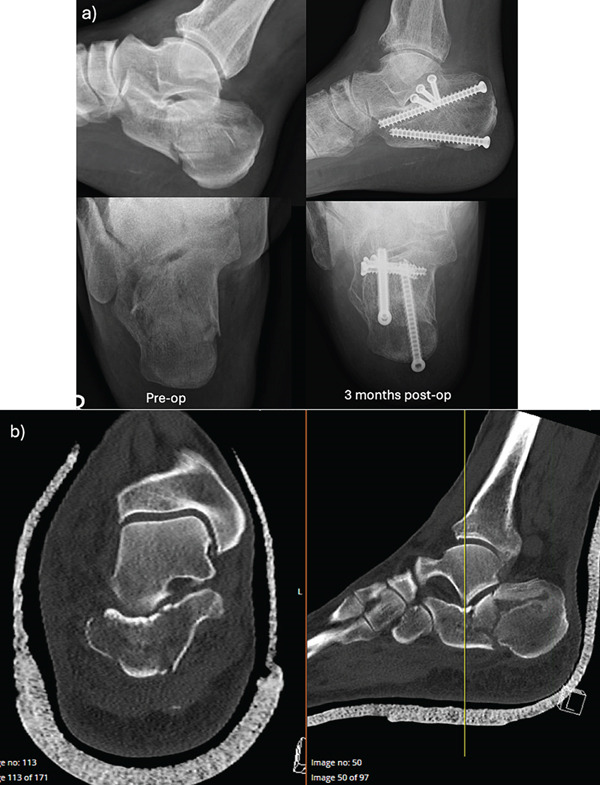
Case C. (a) Pre‐ and postoperative x‐rays. Intraoperative x‐rays unable to be retrieved. (b) Preoperative CT scan of a closed, right‐sided Sanders 2B calcaneus fracture.
